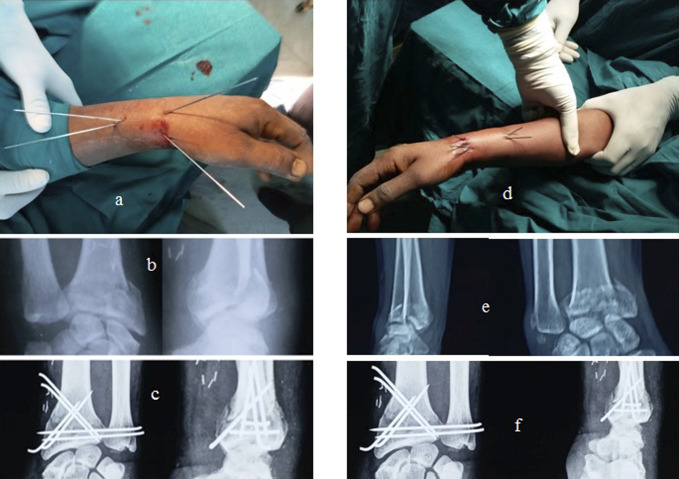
Photograph showing pinning of fractures with coronal fragments. A, Modification of the plane of the triangles. B, Showing the coronal fragments. C, Post-op radiograph showing the orientation of the k-wires. D, Additional k-wire set for engaging the coronal split. E, Pre-op radiograph showing the coronal split. F, Post-op radiograph showing the orientation of the k-wires.
